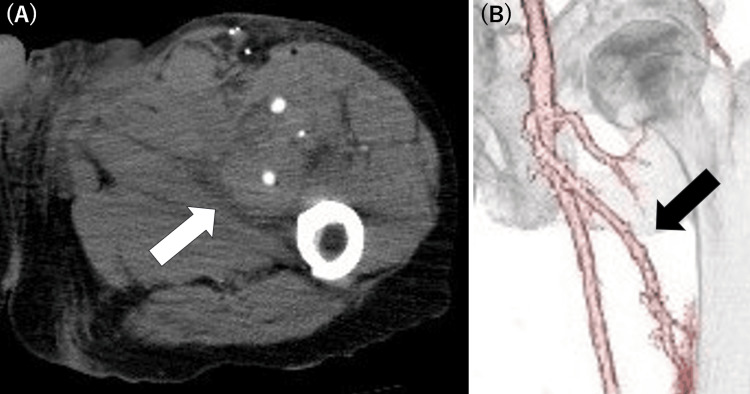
Postoperative CECT images (A) Axial view showing the patent graft (white arrow). (B) Three-dimensional reconstruction demonstrating successful bypass with good graft patency (black arrow). CECT: contrast-enhanced computed tomography
